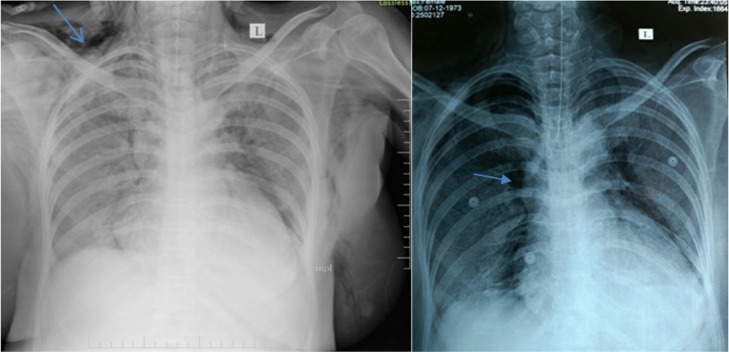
Chest X-ray showing subcutaneous emphysema and pneumomediastinum.
